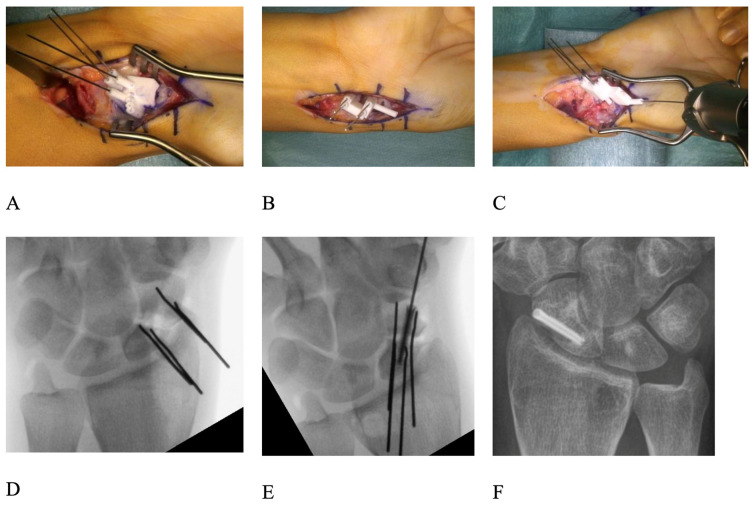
Clinical images of: (A): First guide to place the K-wires in the scaphoid and remove the dorsal overlap by drilling. (B): Reposition guide with now parallel K-wires. (C): Additional guide to place the guidewire for the cannulated screw. (D): Fluoroscopy view of the reposition. (E): Fluoroscopy view of the cannulated screw in situ (F): X-ray 6 months postsurgery with consolidation of the scaphoid.
